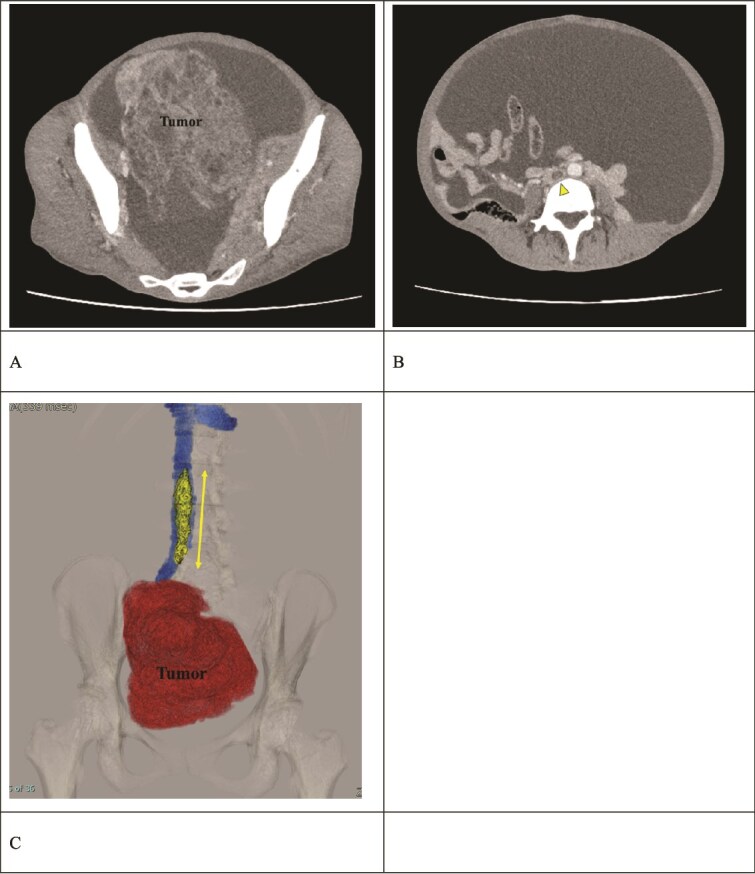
Preoperative contrast-enhanced CT. (A) A right ovarian tumor is observed in the pelvic cavity. (B) Massive ascites and a thrombus (arrow) in the IVC are observed. (C) The 3D reconstruction image shows a venous thrombus (arrow) extending from the right iliac vein to the IVC at the L2 level. The distance between the renal vein and the thrombus tip is only 25 mm.
